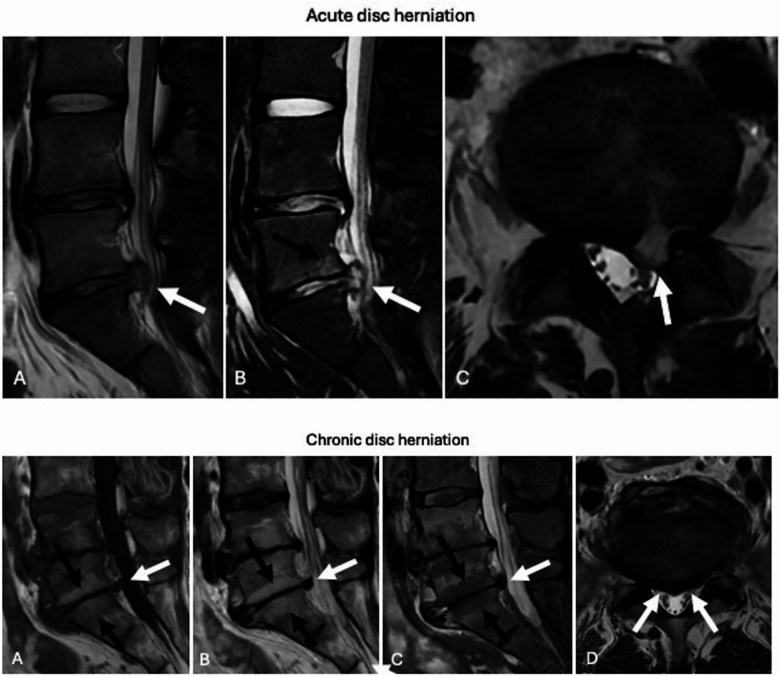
Acute L5-S1 disc herniation (white arrows) with high signal on sagittal T2-weighted (A), sagittal STIR (B), and axial T2-weighted (C) images, due to the high degree of hydration. No reactive osteophyte is observed, while it is possible to see bone edema of L5 close to the herniated disc on sagittal STIR image (B, black arrow). Chronic L5-S1 disc herniation (white arrows) with low signal on sagittal T1-weighted (A), sagittal T2-weighted (B), sagittal STIR (C), and axial T2-weighted (D) images, due to the low degree of hydration. Reactive osteophyte is not associated with bone edema. Modic 2 fatty changes (black arrows) of the bone adjacent to the endplates is observed with high signal on T1-weighted (A) and T2-weighted (B) images, and low signal on STIR (C)
